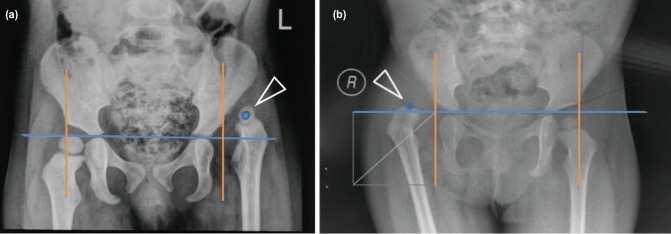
(a) One example of AP pelvic radiograph with grade 4 Tonnis classification and (b) grade 4 IHDI classification. From the radiograph it can be seen that the ossific nucleus of the right femoral head is not visible on the radiograph.
